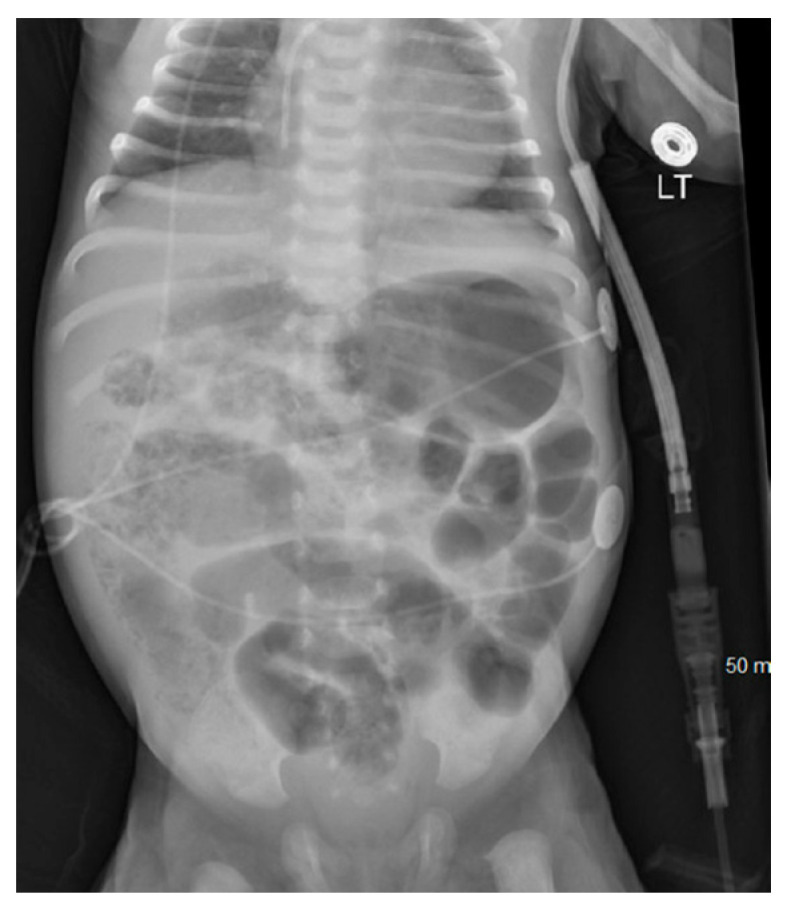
Day 25 abdominal X-ray showing pneumatosis.
